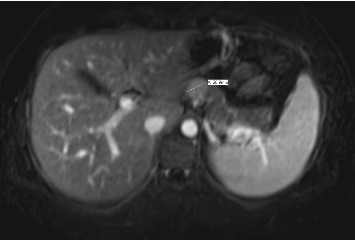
MRI of the abdomen: 2.1 cm slightly T2 hyperintense, T1 hypointense nodule in the pancreatic neck.
